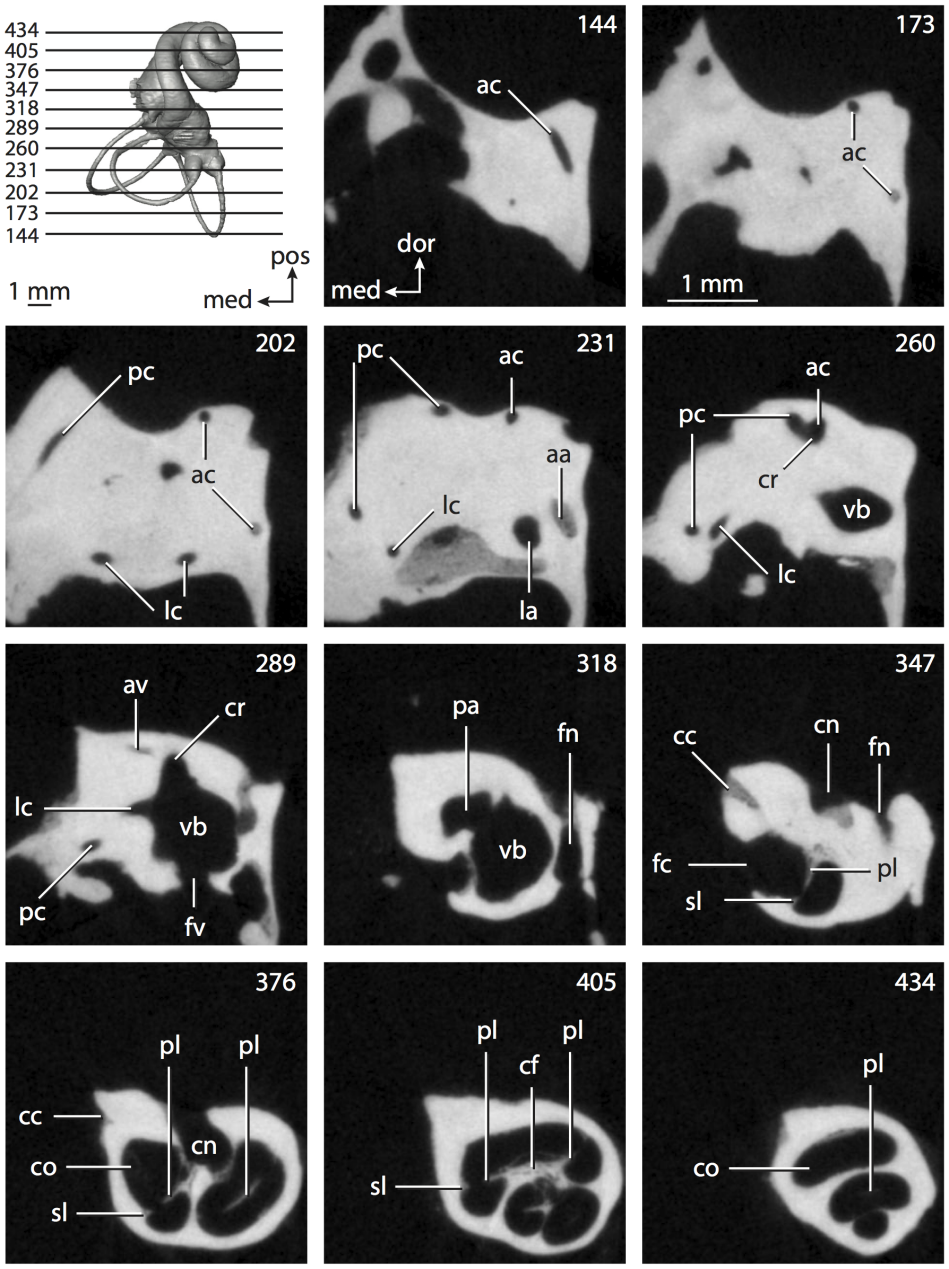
CT slices through ear region of*Dasypus novemcinctus.* Abbreviations listed at the end of the Materials and Methods section.
